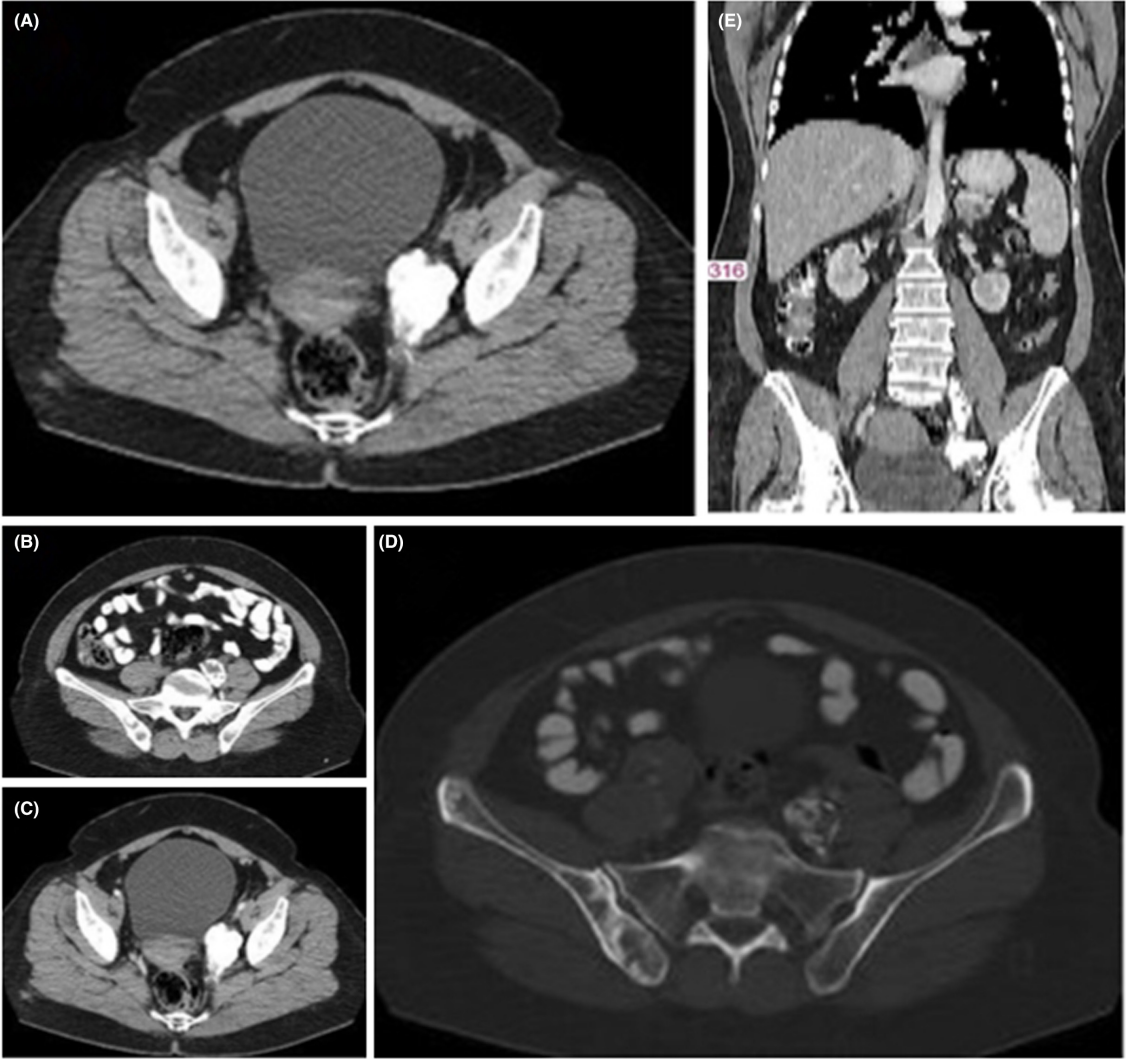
(A and B) noncontrast‐enhanced axial CT images exhibit a heterogeneous retroperitoneal mass with extensive dense popcorn‐like calcifications in the left paracervical area and the left common iliac vein. (C and D) Postcontrast images reveal only subtle heterogeneous enhancement of the mass mainly in its periphery. The lytic bony lesions are identified in the left iliac bone, indicative of metastasis. (E) Postcontrast coronal CT clearly displays the extension of the mass to the ipsilateral distended external, internal and common iliac veins.
